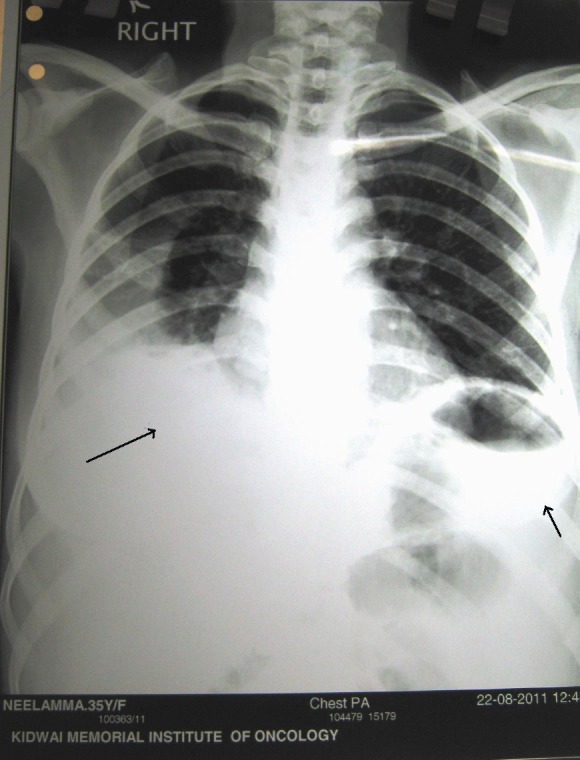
Post-operative chest X-ray showing bilateral pleural effusion right more than left (following pleural tapping)
